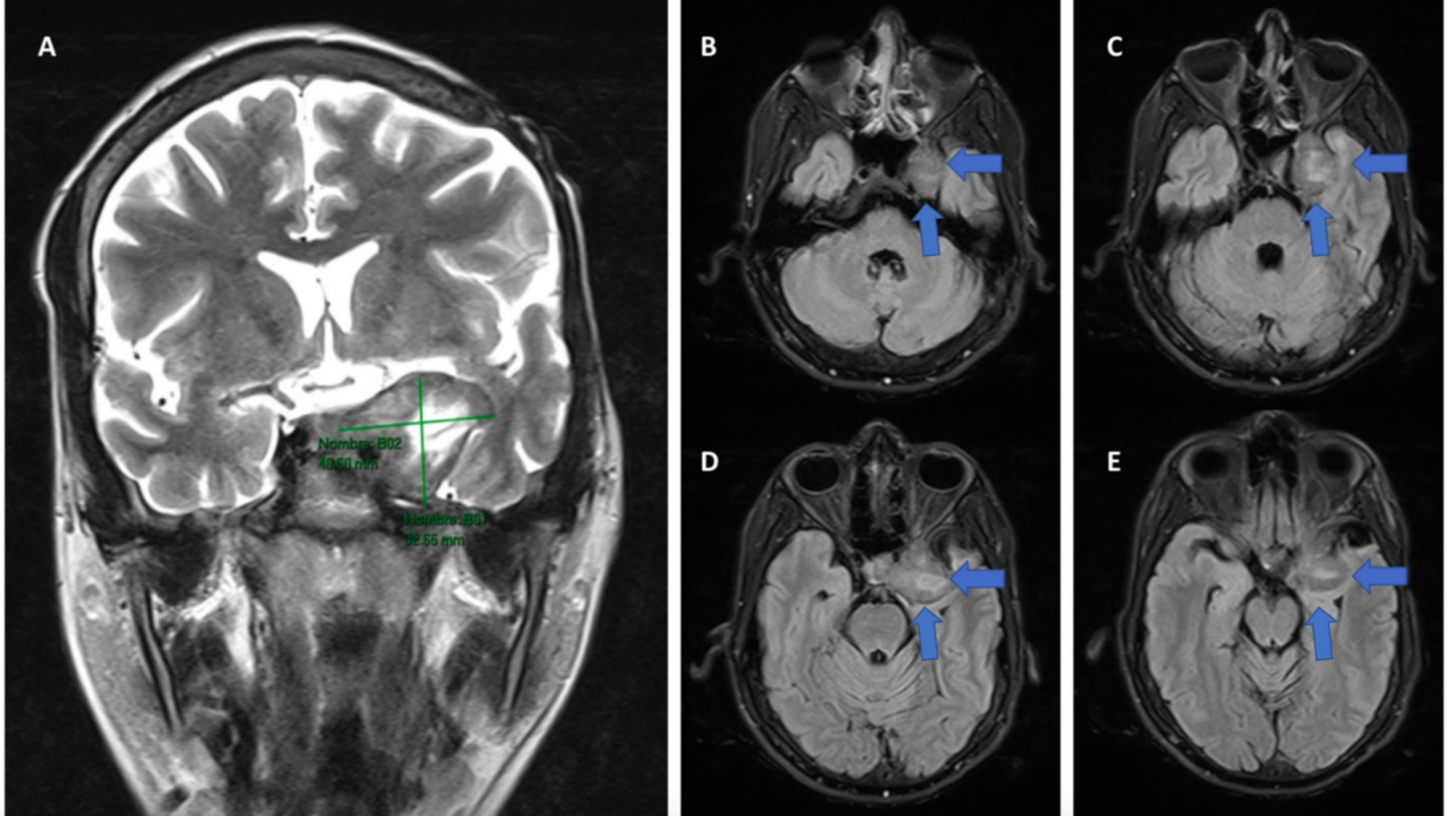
Cerebral MRI (A) Coronal section showing the dimensions of the tumor. (B) Axial section of the lower part of the tumor. (C) Axial section where the relationship of the mass with the skull base structures begins to be shown. (D) Axial section showing how the mass infiltrates the cavernous sinus. (E) Axial section of the upper part of the tumor.
